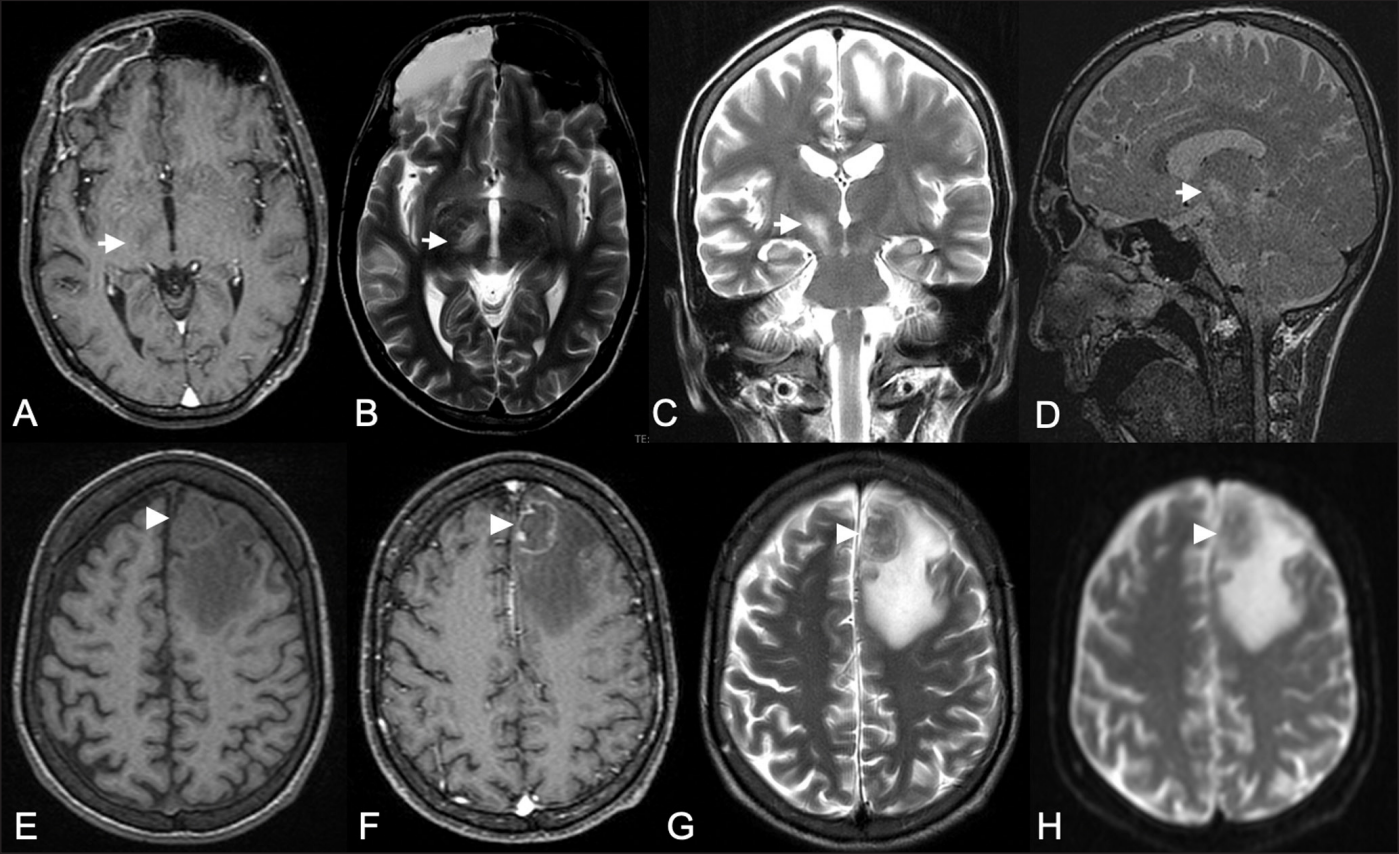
Cranial magnetic resonance imaging of the patient at presentation. A non-enhancing hypointense focus (white arrow) on postcontrast T1 weighted imaging (A) is seen on the right subthalamic region and cerebral peduncle appearing hyperintense on axial (B), coronal (C) and sagittal (D) T2 weighted images, representing focal cerebritis. Also seen is an abscess formation in the left frontal region (white arrowhead) with a slightly hyperintense rim on noncontrast T1 weighted image (E) suggestive of tuberculoma. On postcontrast axial T1 weighted image (F), it appears rim-enhancing with a nodular area of enhancement along its medial wall possibly an “eccentric target sign”, with a hypointense core and surrounding moderate vasogenic edema on axial T2 weighted (G) and diffusion weighted (H) imaging. Considerations for this space-occupying lesion include a tuberculoma and cerebral toxoplasmosis.
